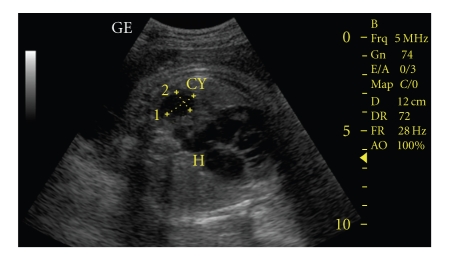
Sonographic examination of congenital cystic lung lesion after thoracocentesis.
